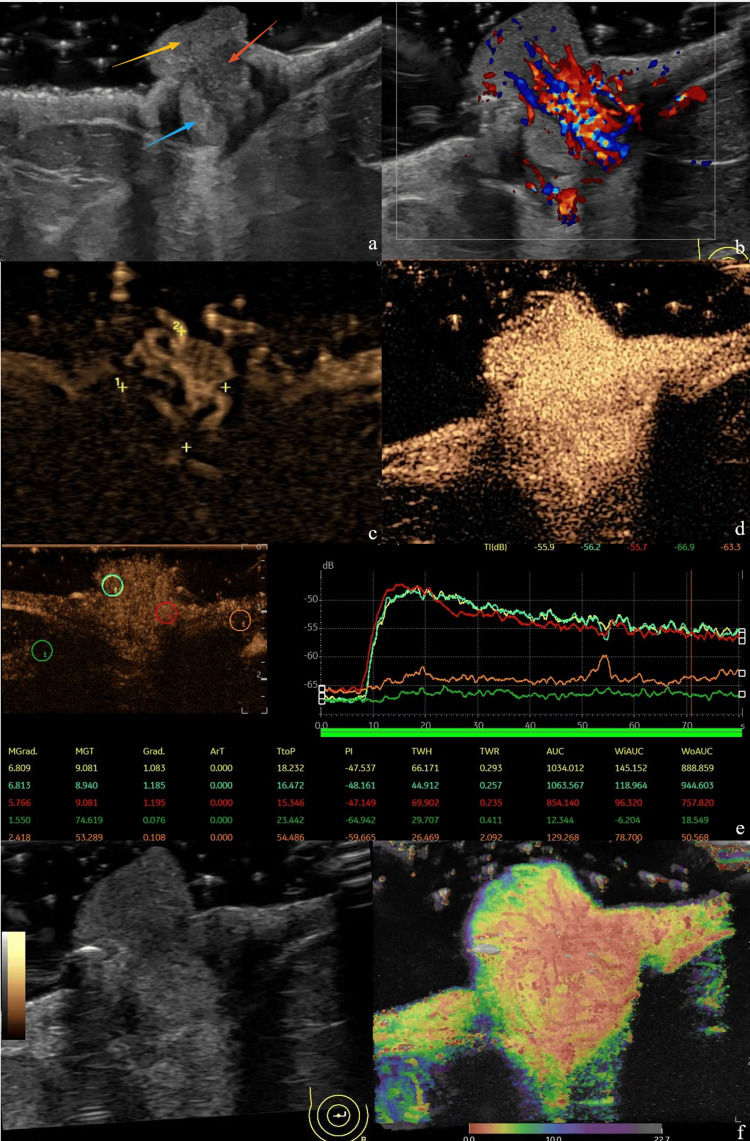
The ultrasound image showing the cross-section of the subpapillary lesion a. Two-dimensional ultrasound image: It can be seen that the slightly lower echo zone, the isoechoic zone and the neoplasms at the nipple seem to form a whole. The red arrows indicate the slightly lower echo zone, the blue arrows indicate the isoechoic zone, and the yellow arrows indicate the protruding papillae. b. CDFI: The lesion at the right nipple is rich in bundle blood flow signals, with Alder grade 3. c. B-Flow condition: The longitudinal section of the nipple reveals a quasi-circular isoechoic region, suggesting that certain sections exhibit a clear boundary between this area and the neoplasms located at the nipple. At the ninth second of contrast-enhanced ultrasound, a concentric high enhancement was observed, and the lesion area increased following enhancement. d-e. CEUS: At the ninth second after the contrast agent was injected through the anterior elbow vein in contrast-enhanced ultrasound, the lesion area expanded compared with the conventional ultrasound range. The TIC curve analysis shows that the enhancement amplitude and pattern of the protruding part of the nipple and the lesion tissue beneath the nipple are comparable. f. MVI: Ultra-micro blood flow imaging using the Sonazoid contrast agent reveals that micro-blood flow is directed from the peripheral tissue toward the center of the lesion, subsequently dispersing from the center to the periphery. Additionally, the curvature of the blood vessels remains regular.
